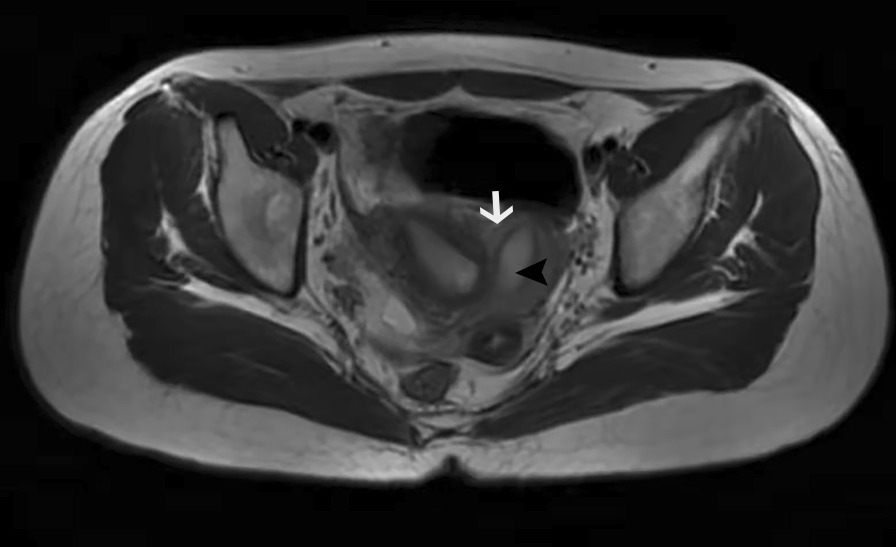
MRI image showing the left uterine cavity (black arrow) communicating with the cervix and irregular fundus(white arrow)
